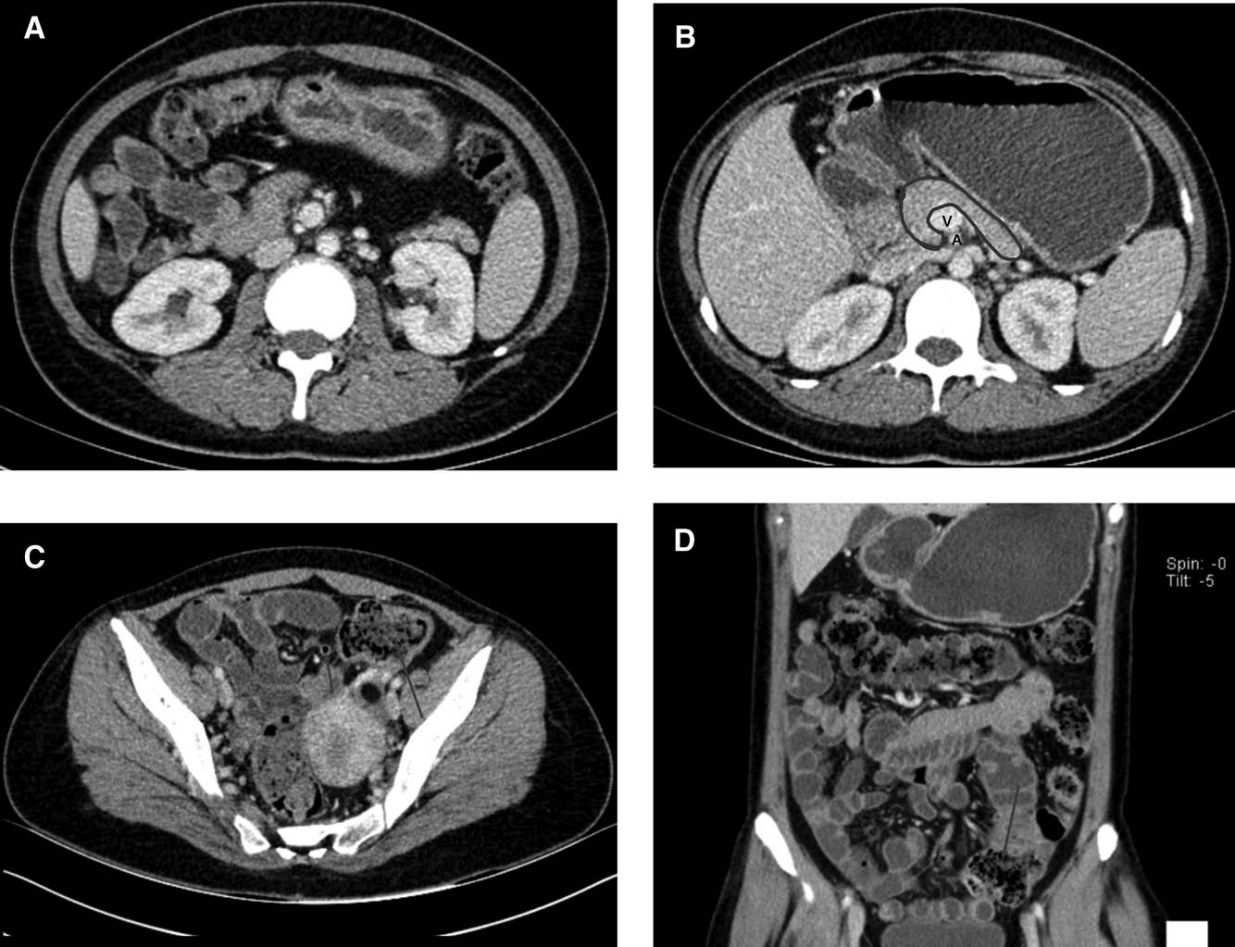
CT image. (A). Axial image shows the absence of a duodenal path dorsal to the SMA. (B) The shape of the pancreas is demonstrated outlined in purple. Note that the uncinate process is hypoplastic (a small amount of pancreatic tissue was seen posterior to the SMV, which did not extend beyond the left lateral margin of the SMV) in this case and that there is mesenteric vascular inversion with the SMV lying anterior to the SMA. (C) Axial image shows a left side of the midline positioned appendix (short arrow) and the cecum in the left lower abdomen (long arrow). (D) Coronal image shows small bowel on right and colon predominately on left. Note the cecum in the left lower abdomen (arrow). CT = computed tomography, SMA = superior mesenteric artery, SMV = superior mesenteric vein.
